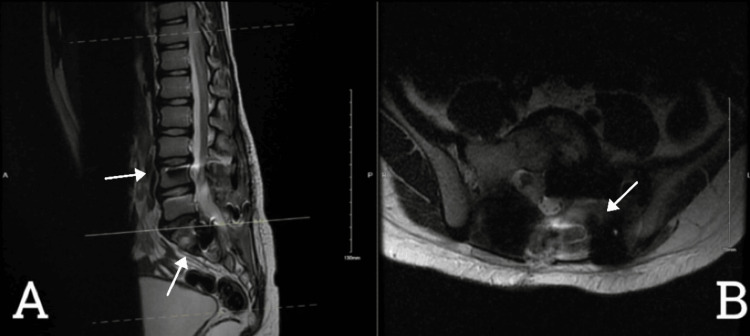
The MRI lumbosacral T2WI sagittal view (A) and axial view (B) The artifact along the area of concern secondary to the screws and rod fixation can be seen in the above panels. Again, evidence of postsurgical changes and the area of the bone graft anterior to the left-most inferior screw can be seen. At a similar level along the lateral sacral crest of S2, there is an area of high T2 and low T1 signal intensity with post-contrast showing mainly thick peripheral enhancement with a central non-enhancing component. The lesion appears to invade the S2 neural foramina. It measures approximately 1.5 × 2.1 cm (AP and transverse dimensions respectively). The remainder of the visualized osseous structures are unremarkable. The visualized spinal cord shows normal morphology and signal intensity.
